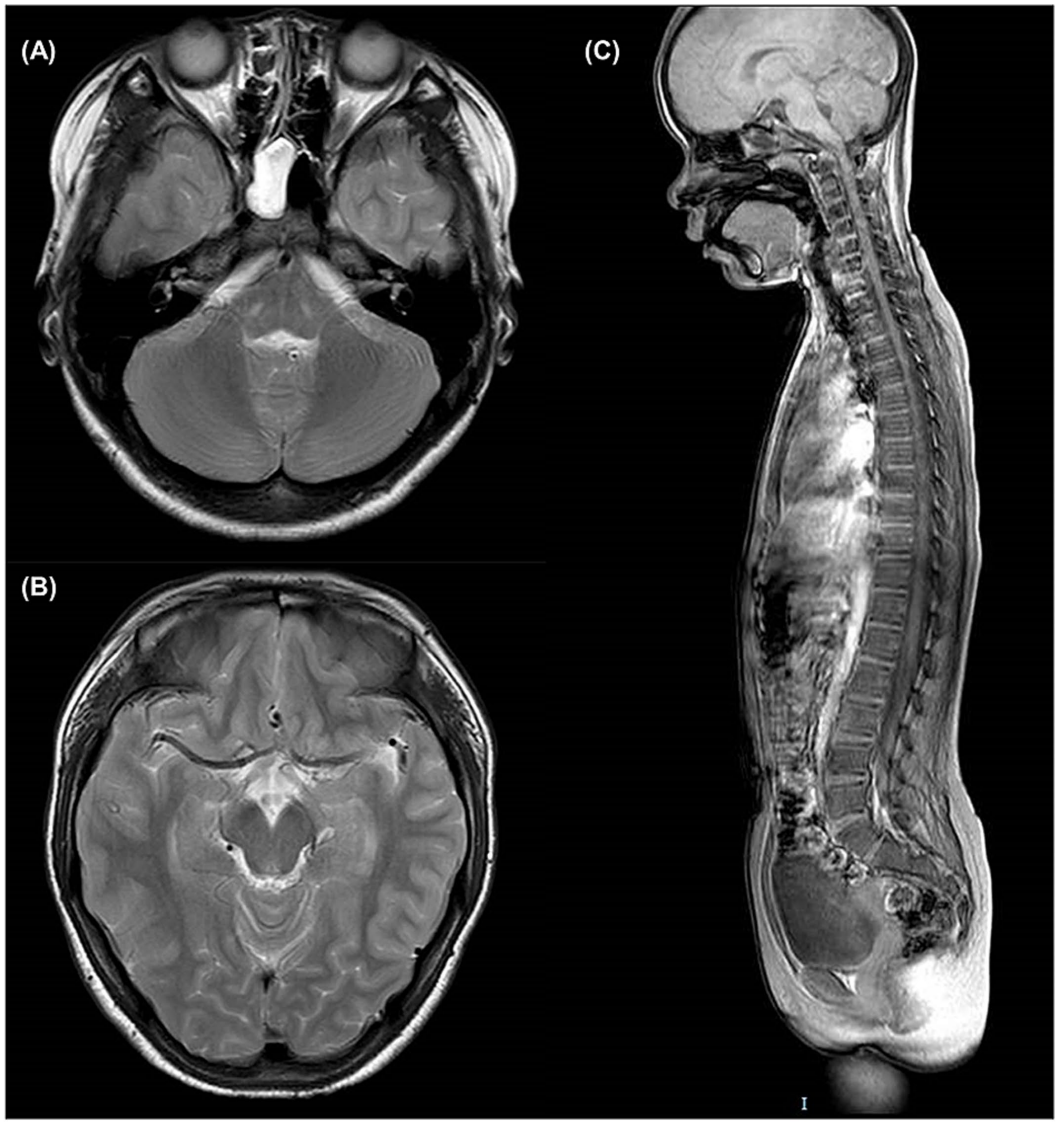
Brain and spinal MRI of patient III2. (A) T2-weighted axial slice at the level of pons. (B) T2-weighted axial slice at the level of mesencephalon. (C) T1-weighted sagittal slice of the entire spine.
